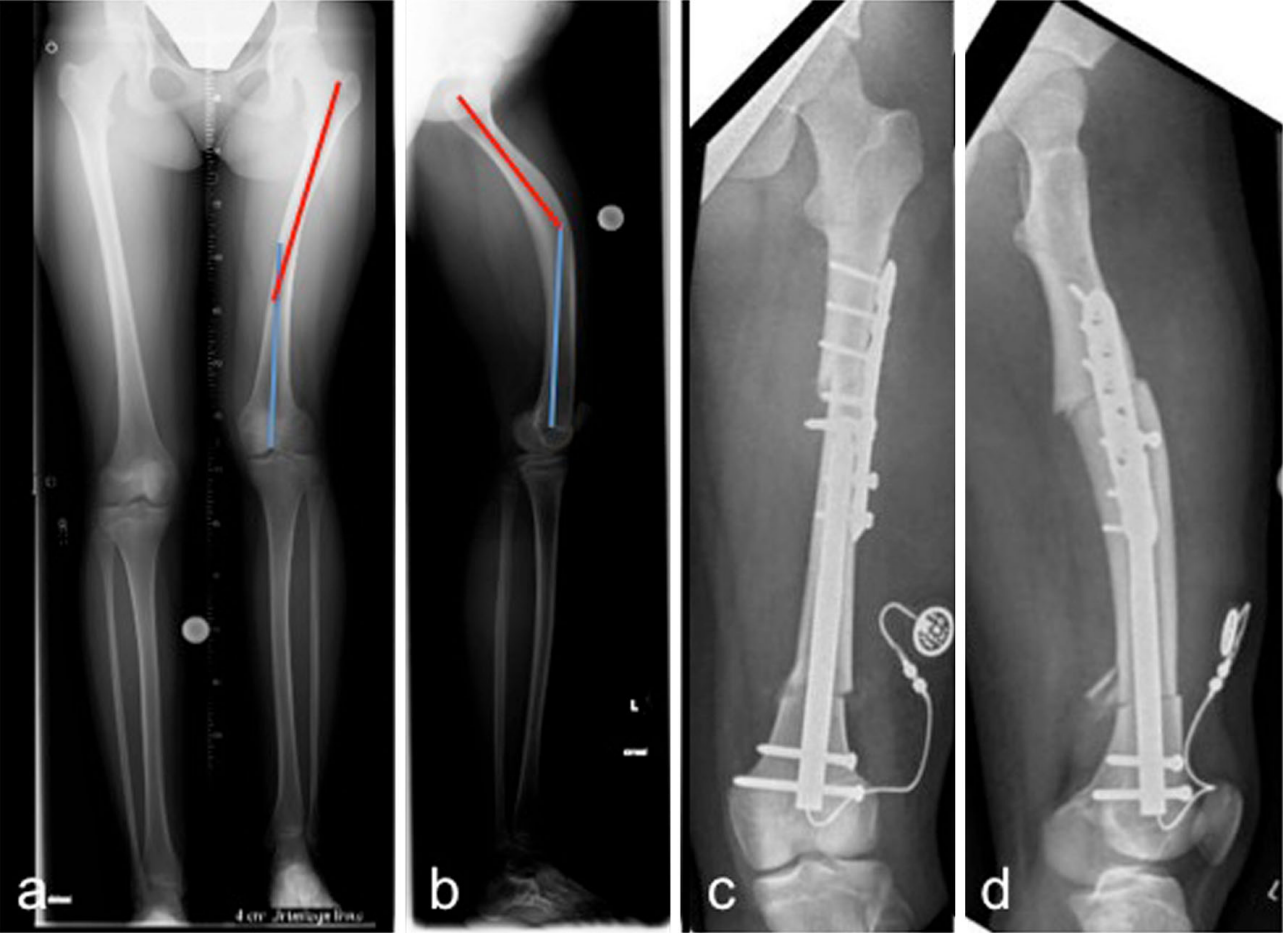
Preoperative anteroposterior (a) and laterolateral (b) long standing radiograph (LSR) of a 15-year-old patient with a combined valgus/flexion deformity and a leg length discrepancy of 4 cm. Respecting the patient’s desire, the use of an external fixator for deformity correction was avoided by performing a second osteotomy at the femoral diaphysis with additional plate fixation. Leg length equalisation was achieved by using the fully implantable motorised lengthening nail (Fitbone^®^) and a lengthening osteotomy at the distal femur. Postoperative result on anteroposterior (c) and laterolateral (d) X-rays
